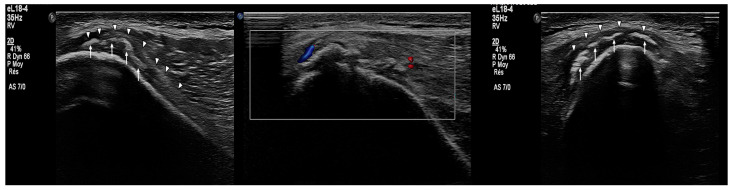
The three-week follow-up ultrasound scans of the posterior iliac crest after the recurrent falls in Case 2: (left) the sagittal plane in B-mode alone, (middle) combined with color Doppler, and (right) the transverse plane, showing periosteal thickening (arrowhead) and calcification (arrow).
